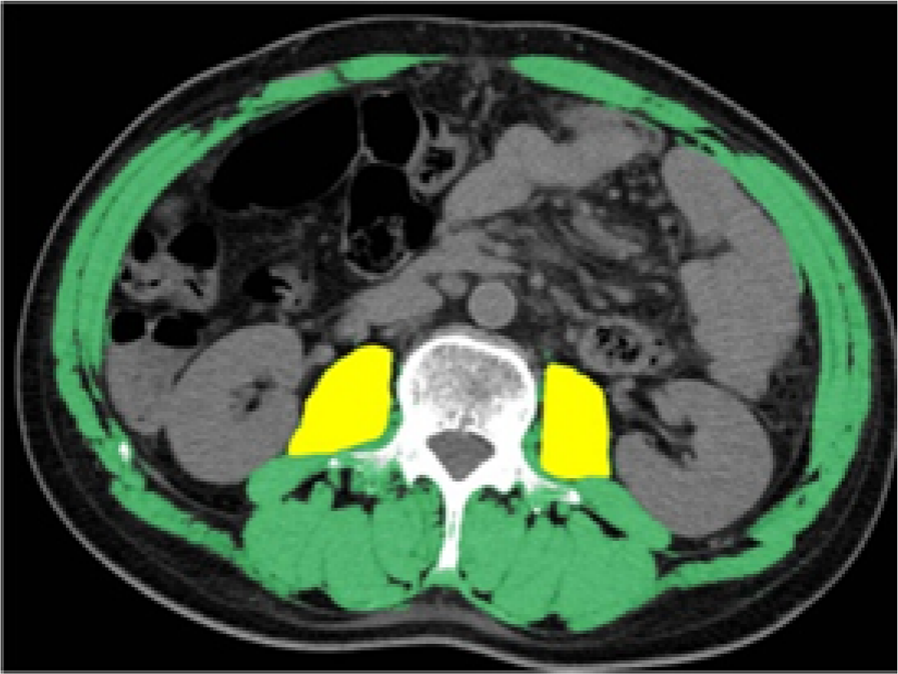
Measurement of skeletal muscle mass at the level of the third lumbar vertebra (L3) with CT images. Green field, total muscle mass; yellow field, psoas muscle mass
